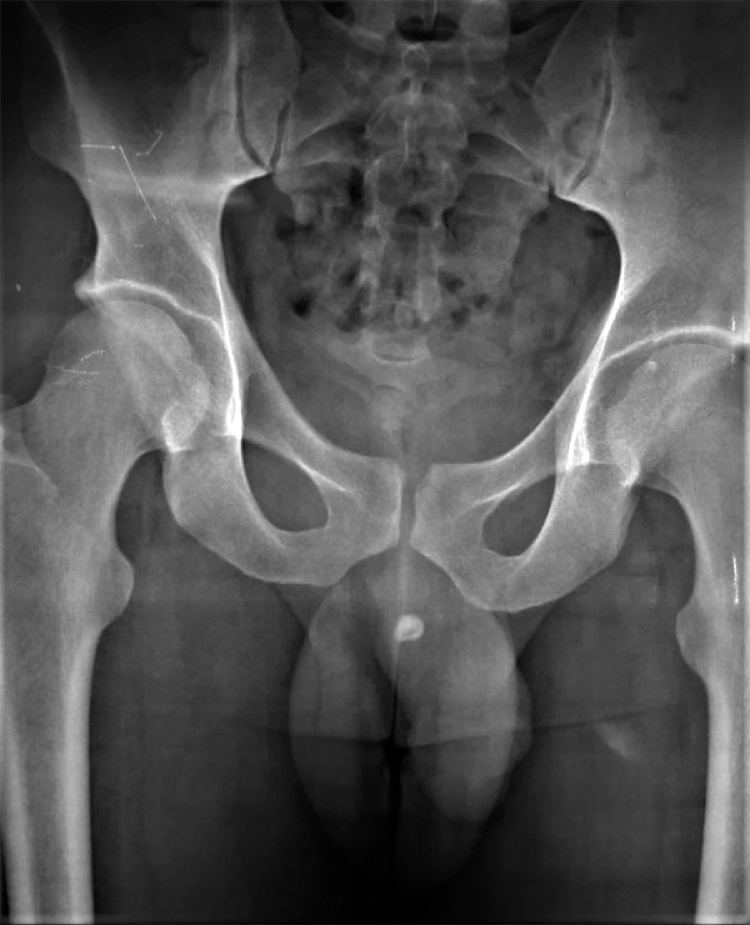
Pelvic X-ray showing the radio-opaque shadow in the penile shaft.
